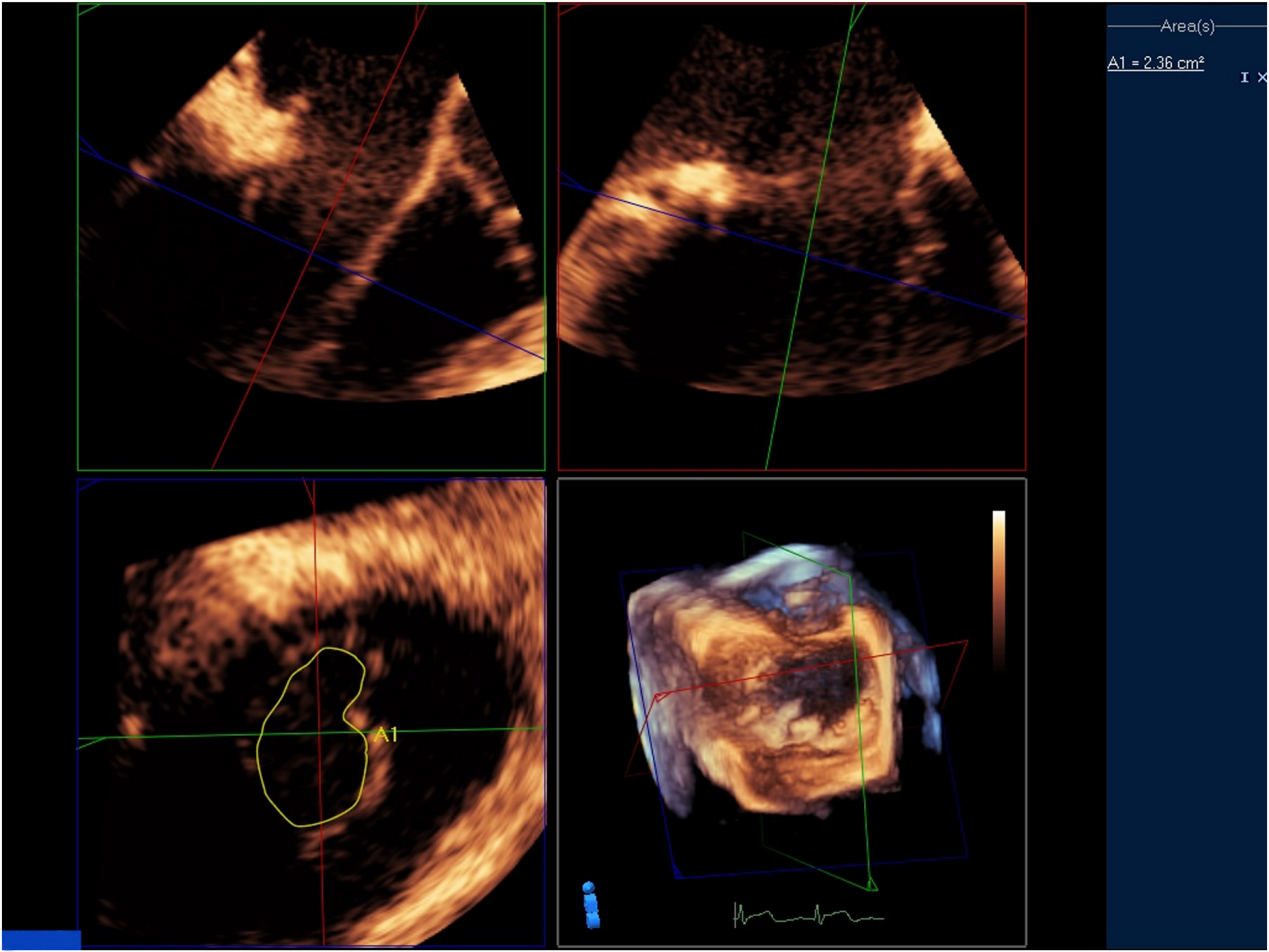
Determination of mitral valve area using 3-dimensional transoesophageal echocardiography.
